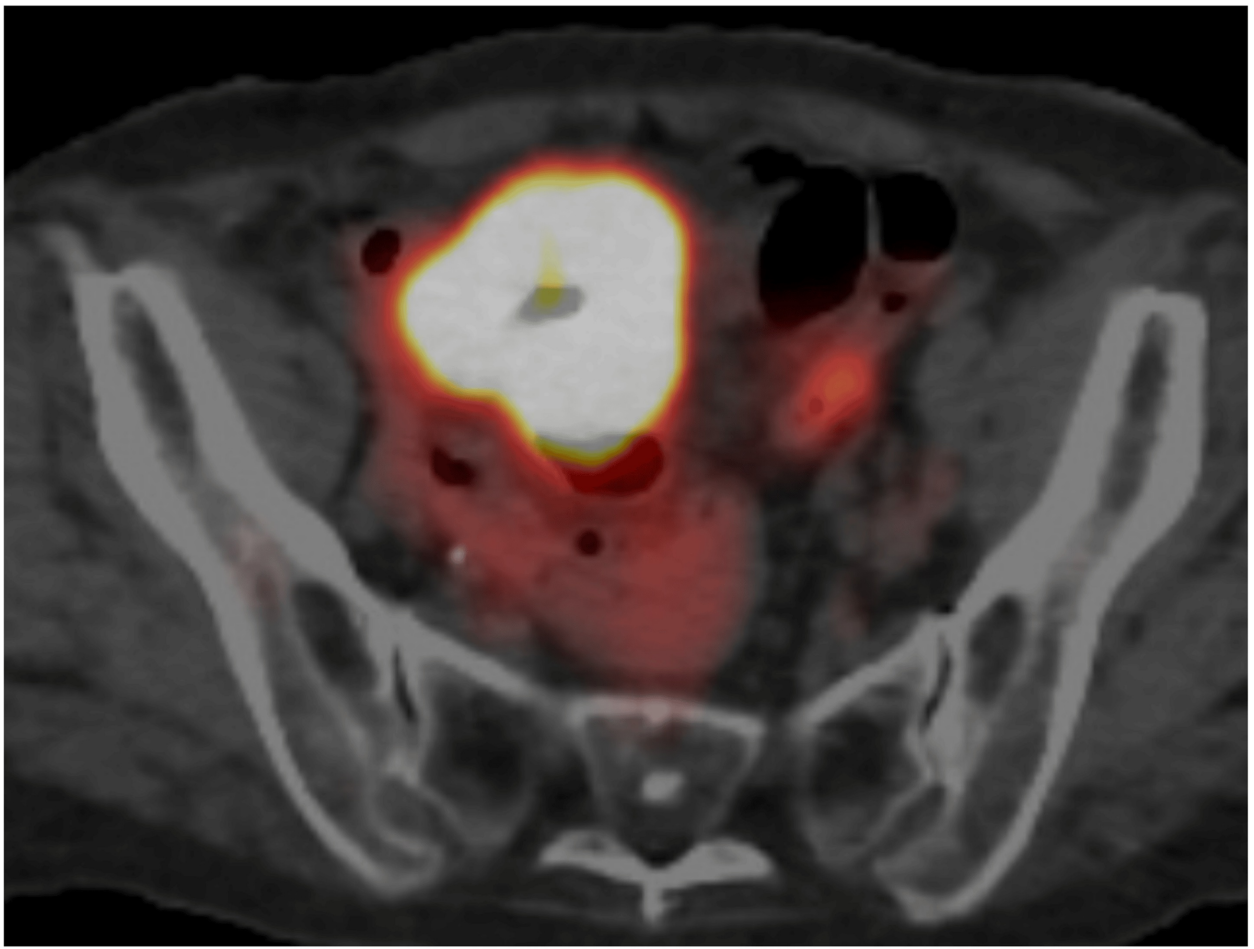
PET/CT findings PET/CT showed avid fluorodeoxyglucose uptake in the mass, especially in the peripheral areas of the mass PET/CT: positron emission tomography/computed tomography
